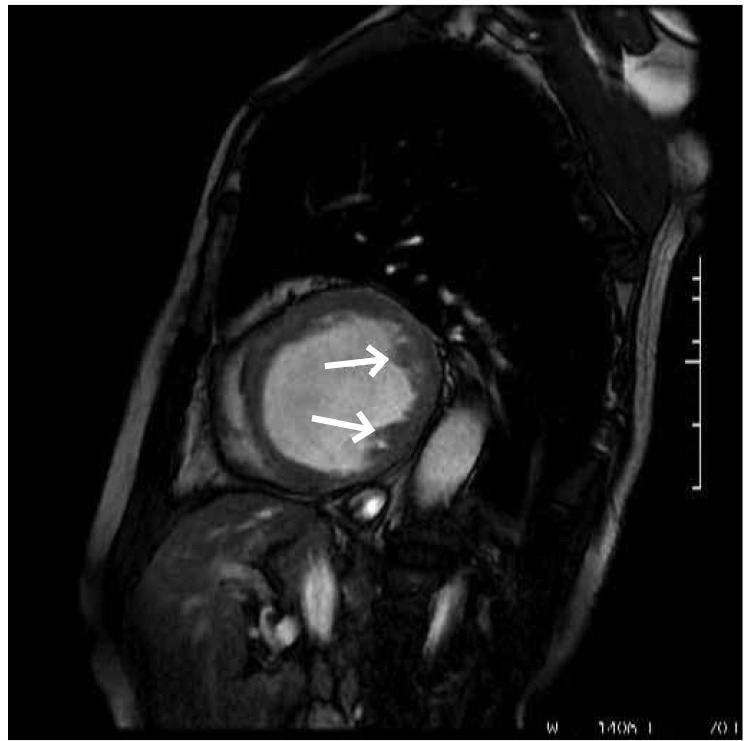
ardiac magnetic resonance imaging confirming the left ventricle aneurysm with intramural thrombus formation (the arrows show the thrombus formation in left ventricle cavity).
